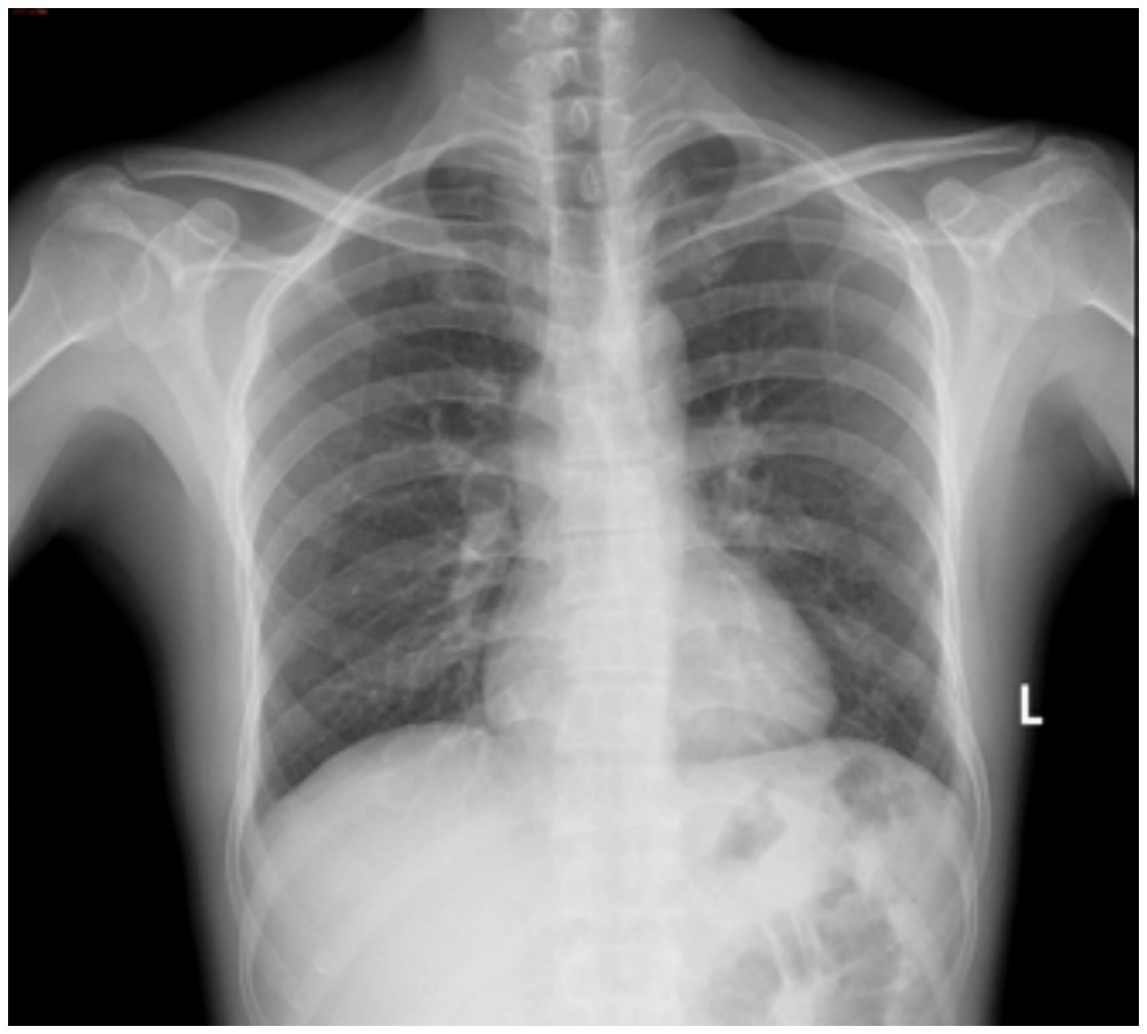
The chest X-ray revealed no significant abnormalities.
